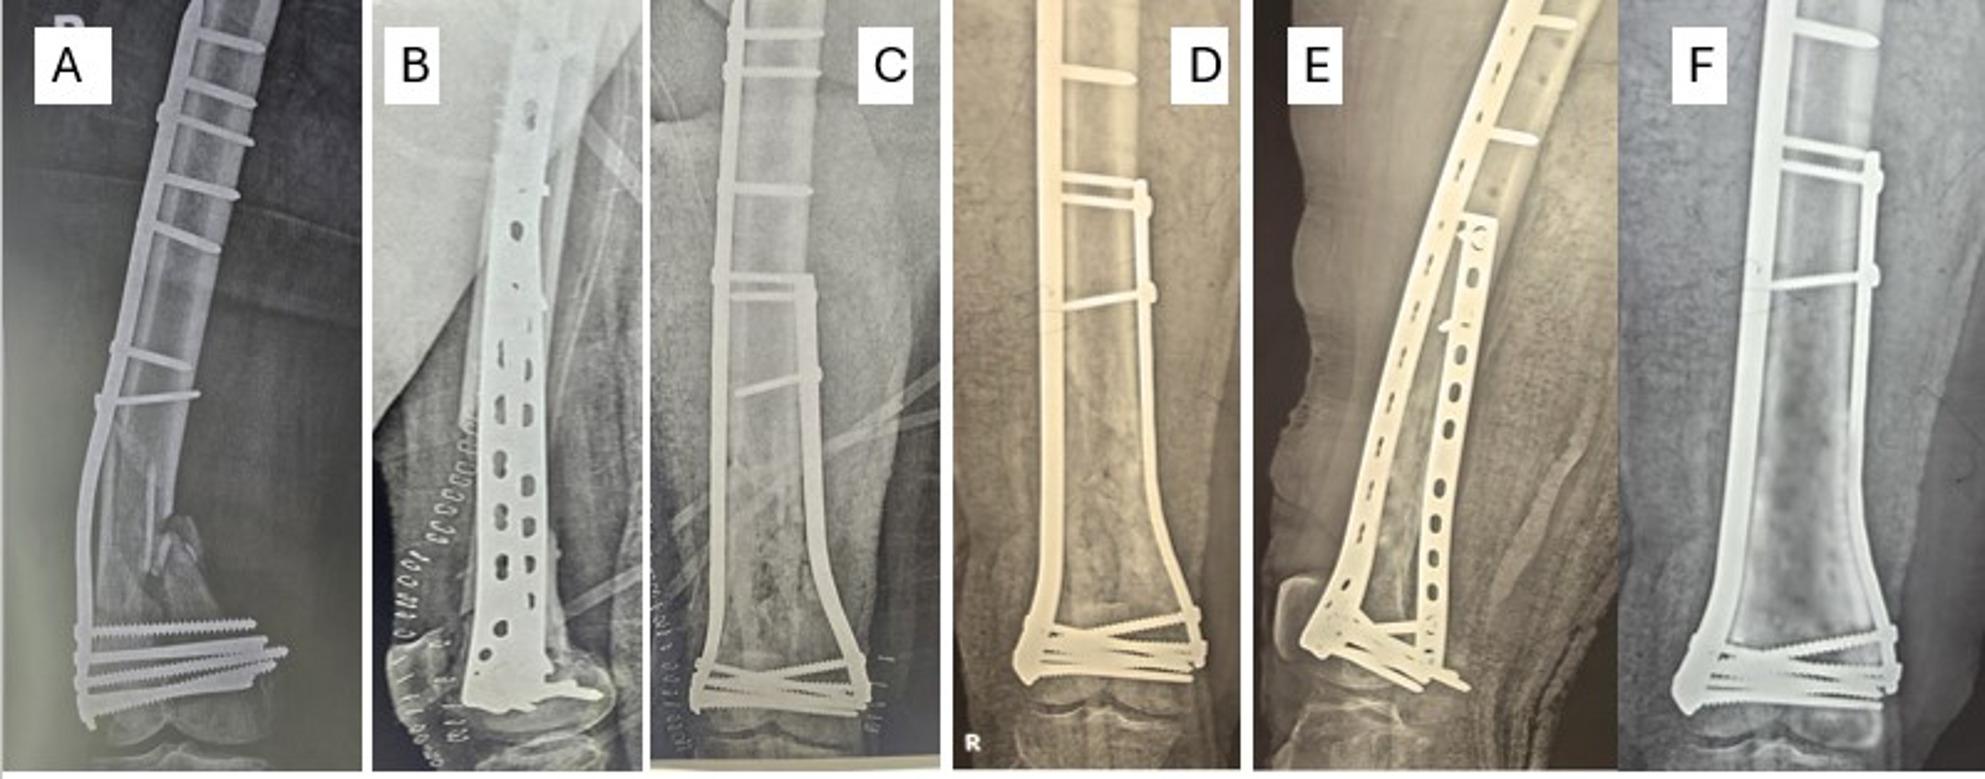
After the patient (Fig. 3) sustained a bent plate A, revision was done by dual plating (locked DFP and contoured narrow DCP), early postoperative radiographs B, C, follow-up at 3 months D, E, and 16-month follow-up with good consolidation of the fracture F
